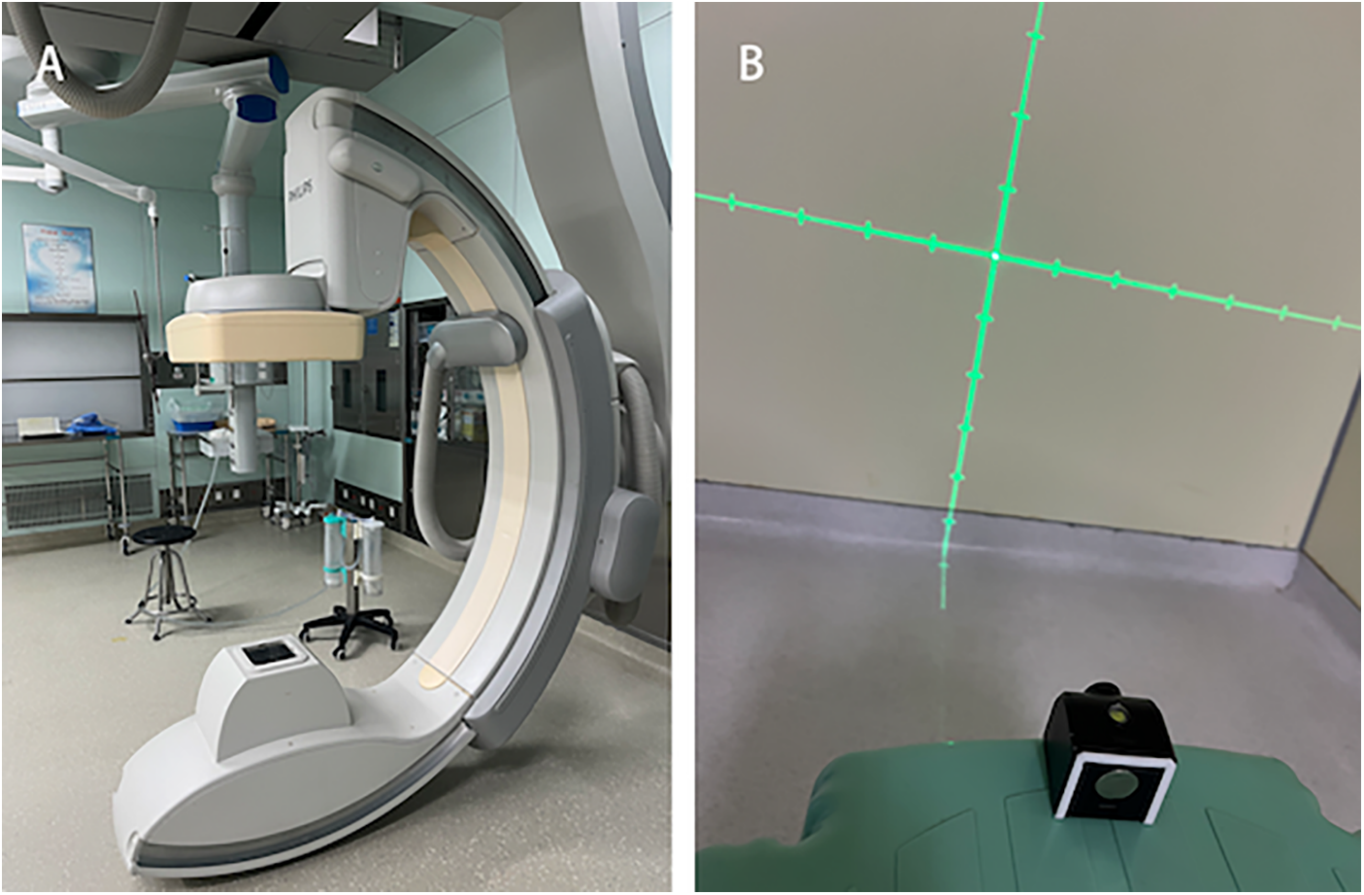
Equipment. (A) C-arm CT equipment (UNIQ FD20, Philips Healthcare, Netherlands). (B) Laser emitter.
